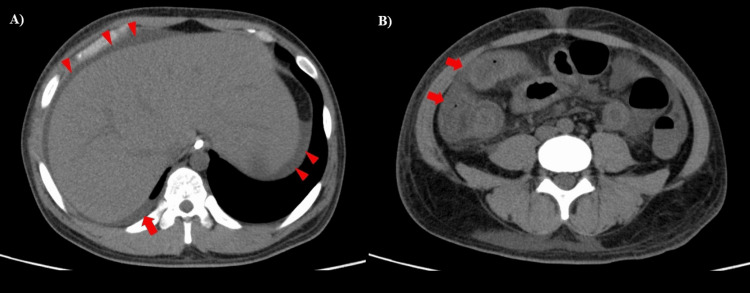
Chest and abdominal computed tomography without contrast enhancement on admission. Chest and abdominal computed tomography without contrast enhancement on admission showed a small amount of right pleural effusion (A, arrow), perihepatic ascites (A, arrowheads), and hepatomegaly. In addition, a thickened intestinal wall extending from the duodenum to the small intestine and ascending colon with increased surrounding fat density was observed (B, arrows).
